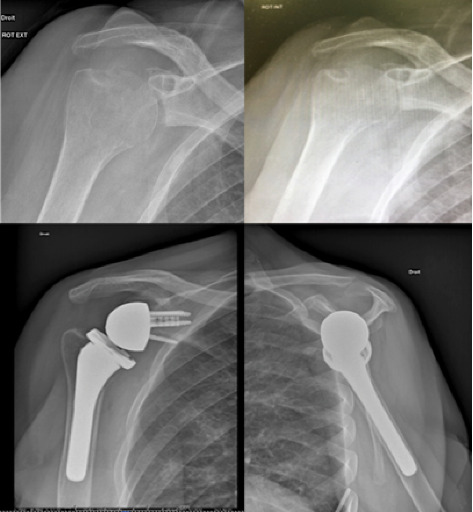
X-ray images before and after surgery.
